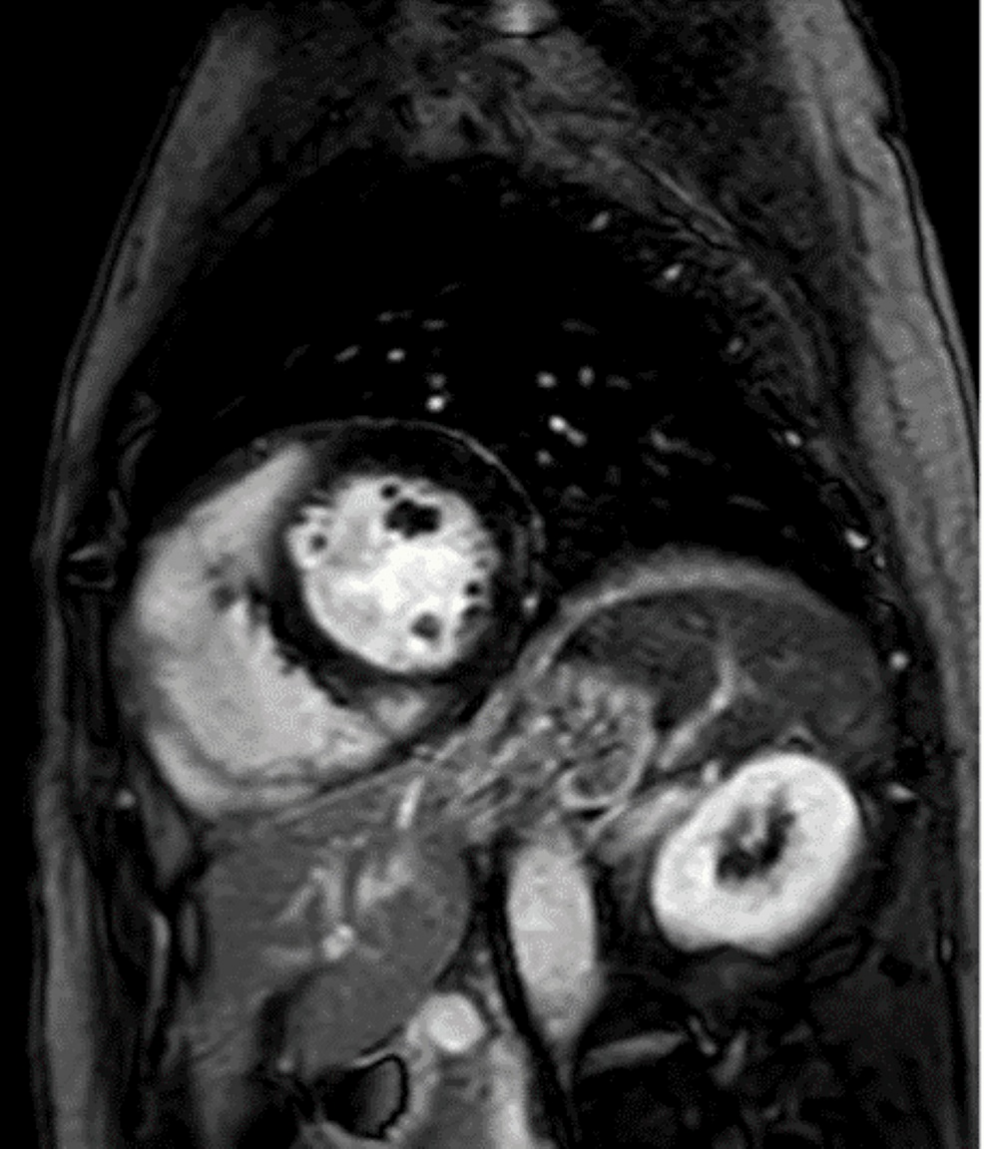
Cardiac MRI (CMR) showing well-demarcated sub-epicardial increased T2 signal suggesting edema in the anteroseptal wall and inferoseptal wall extending from base to apex
